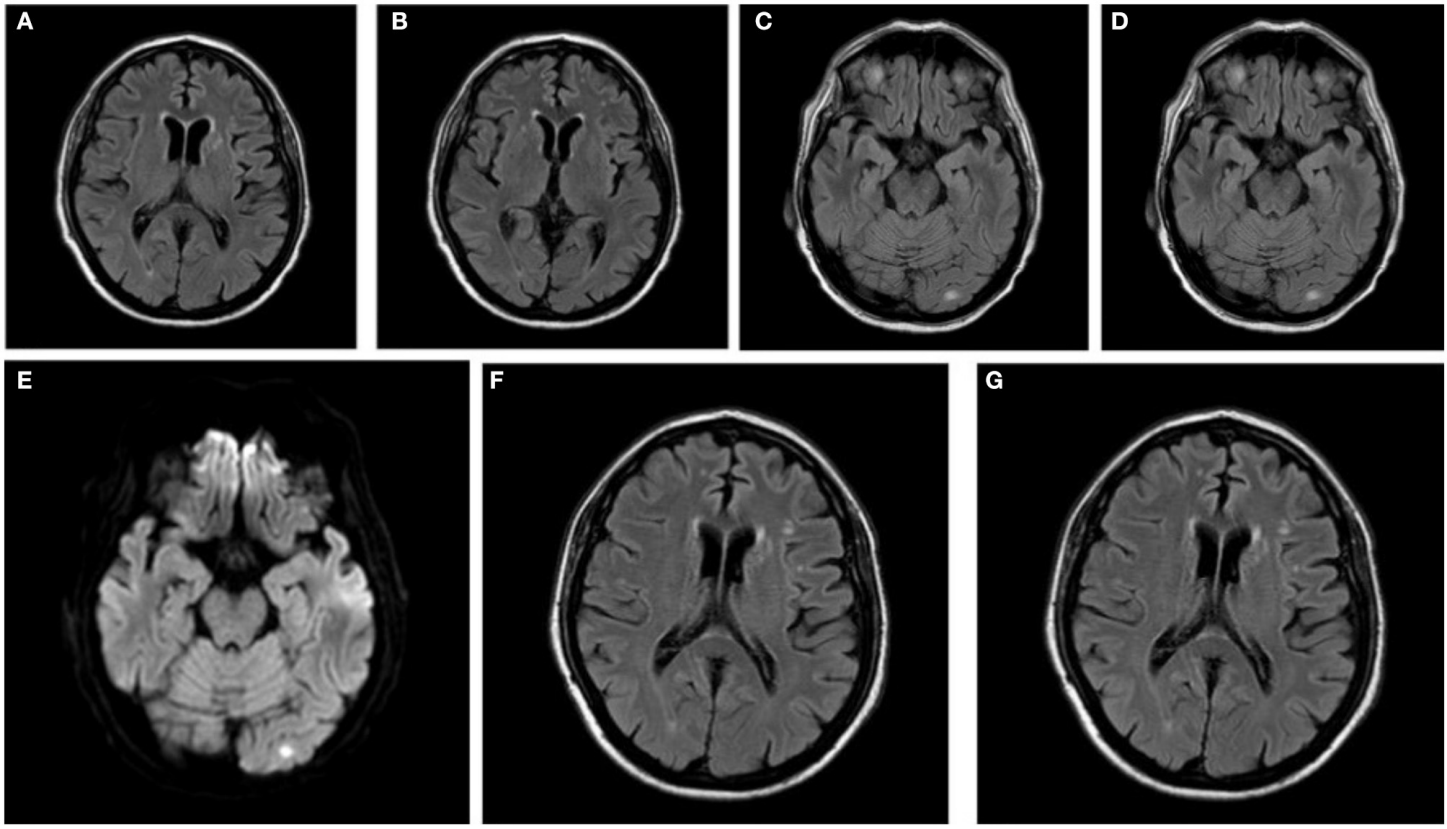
Shoot from brain MRI of May 8 in (A–E): (A,B) show T2-FLAIR acquisition. (C) Shows occipital lesion in T2-FLAIR acquisition. (D) Shows parietal lesion in T2-FLAIR acquisition. (E) Shows occipital lesion positive in DWI. Shoot from Brain MRI of June 16: (F,G) show T2-FLAIR acquisition.
